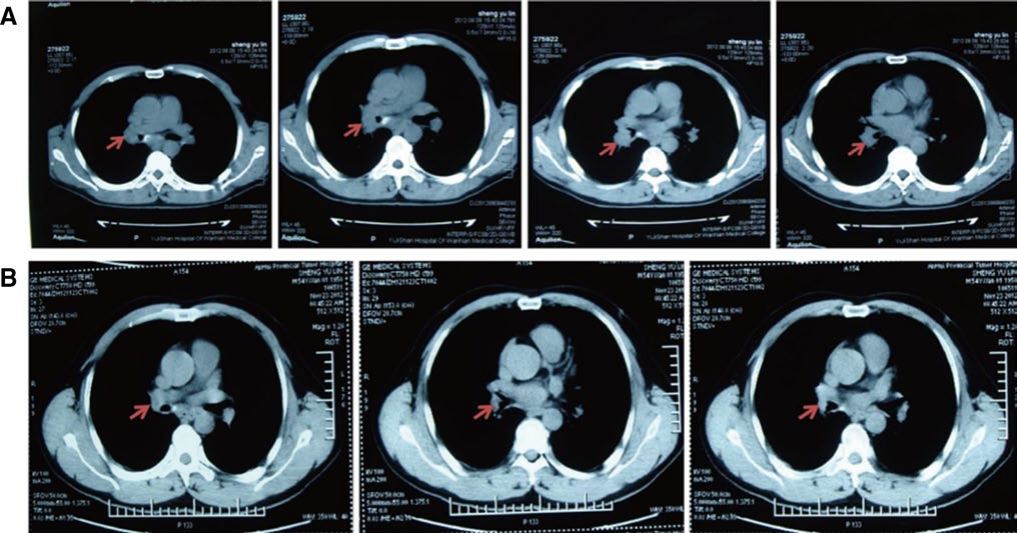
CT scan of the patient with adenocarcinoma of the *right* lung before (a) and after (b) interventional therapy. The *arrows* showed the position of lesions
